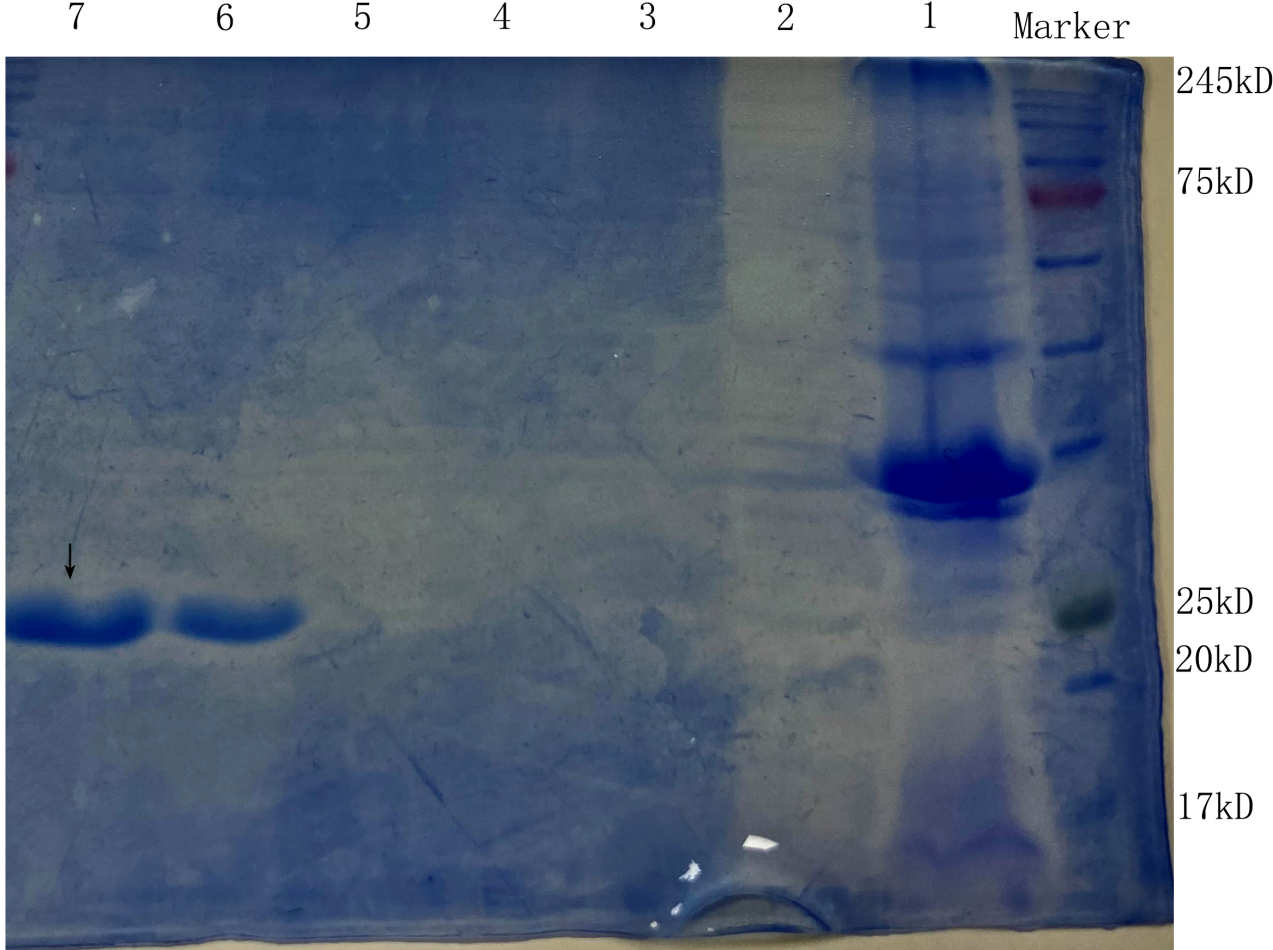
The SDS-PAGE of the anti-restriction protein ArdA. Lanes 1 and 2 are the precipitate and supernatant of *E. coli* BL21 (pET20b-ArdA) obtained by ultrasonic fragmentation without purification, respectively. Lanes 3, 4, 5, 6 and 7 are the target protein ArdA with His tag obtained after purification with 100 mM-500 mM imidazole-eluting nickel columns, located at the positions indicated by arrows.
